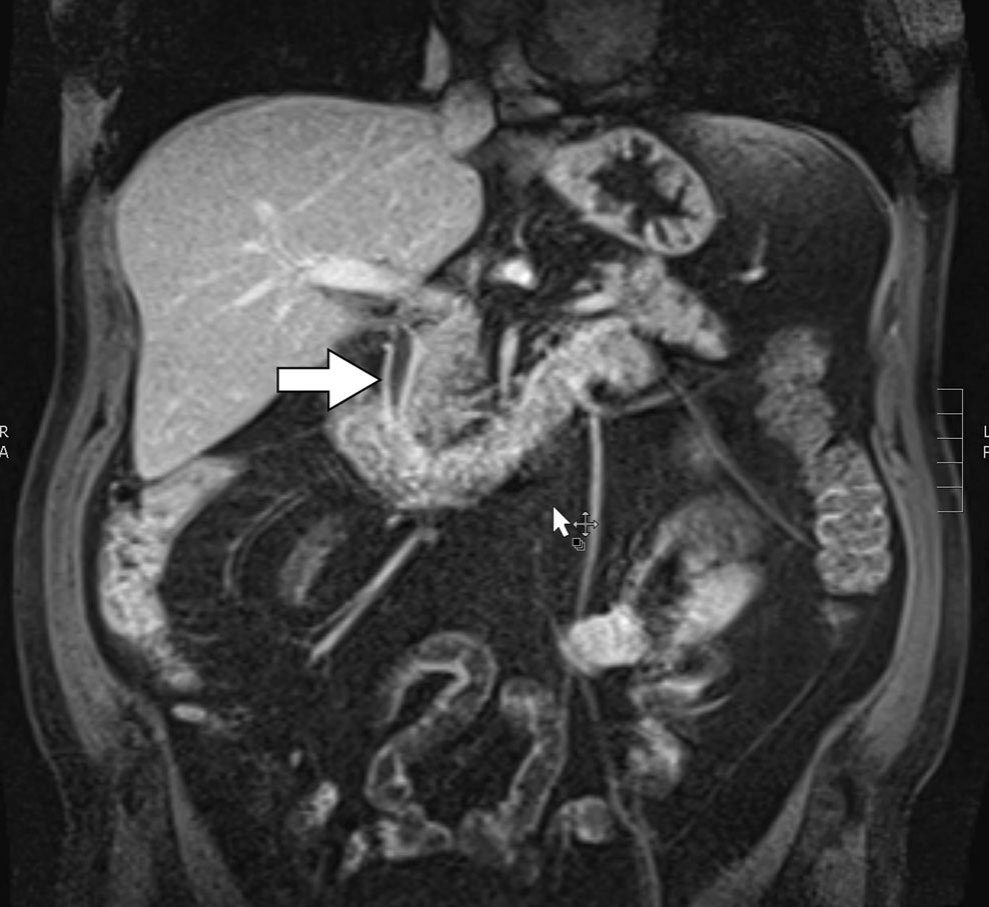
A 39-year-old male with non-Hodgkin’s lymphoma treated with doxorubicin. Coronal post-contrast MR image shows the thickened, enhancing biliary wall (*arrow*) of the common bile duct consistent with biliary inflammation
